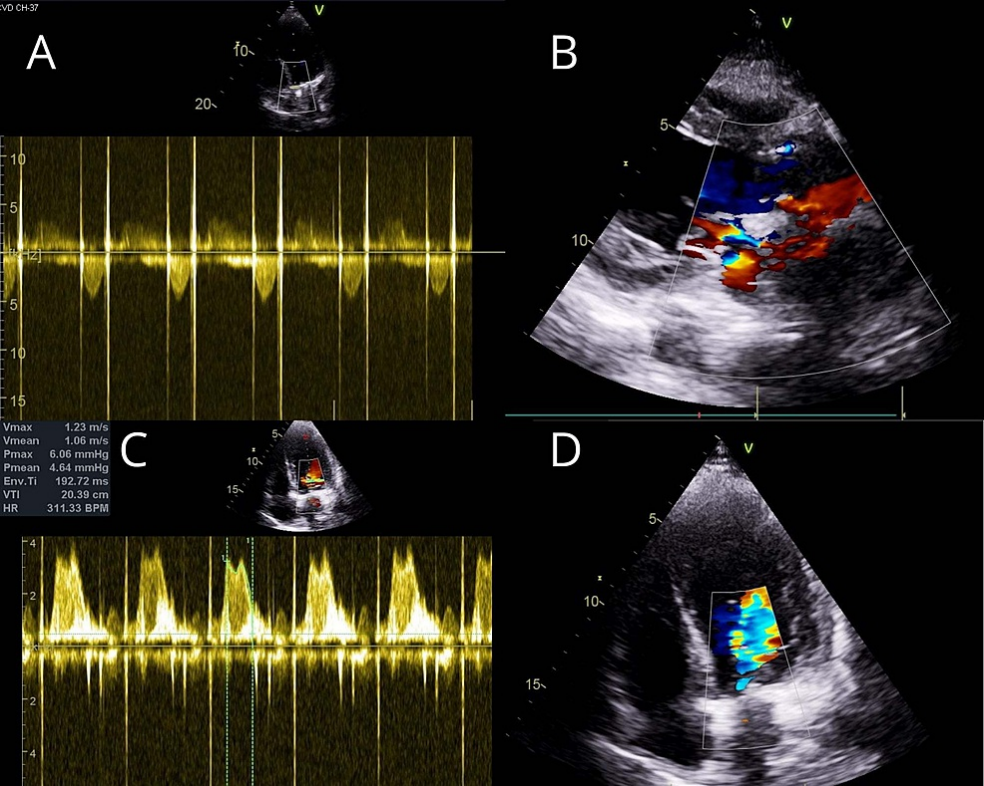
Postoperative transthoracic echocardiography (A) Five-chamber view with continuous-wave Doppler showing no paravalvular leak. (B) Parasternal long-axis view showing no paravalvular leak. (C) Four-chamber view with continuous-wave Doppler showing no residual mitral regurgitation. (D) Four-chamber view showing no mitral regurgitation.
